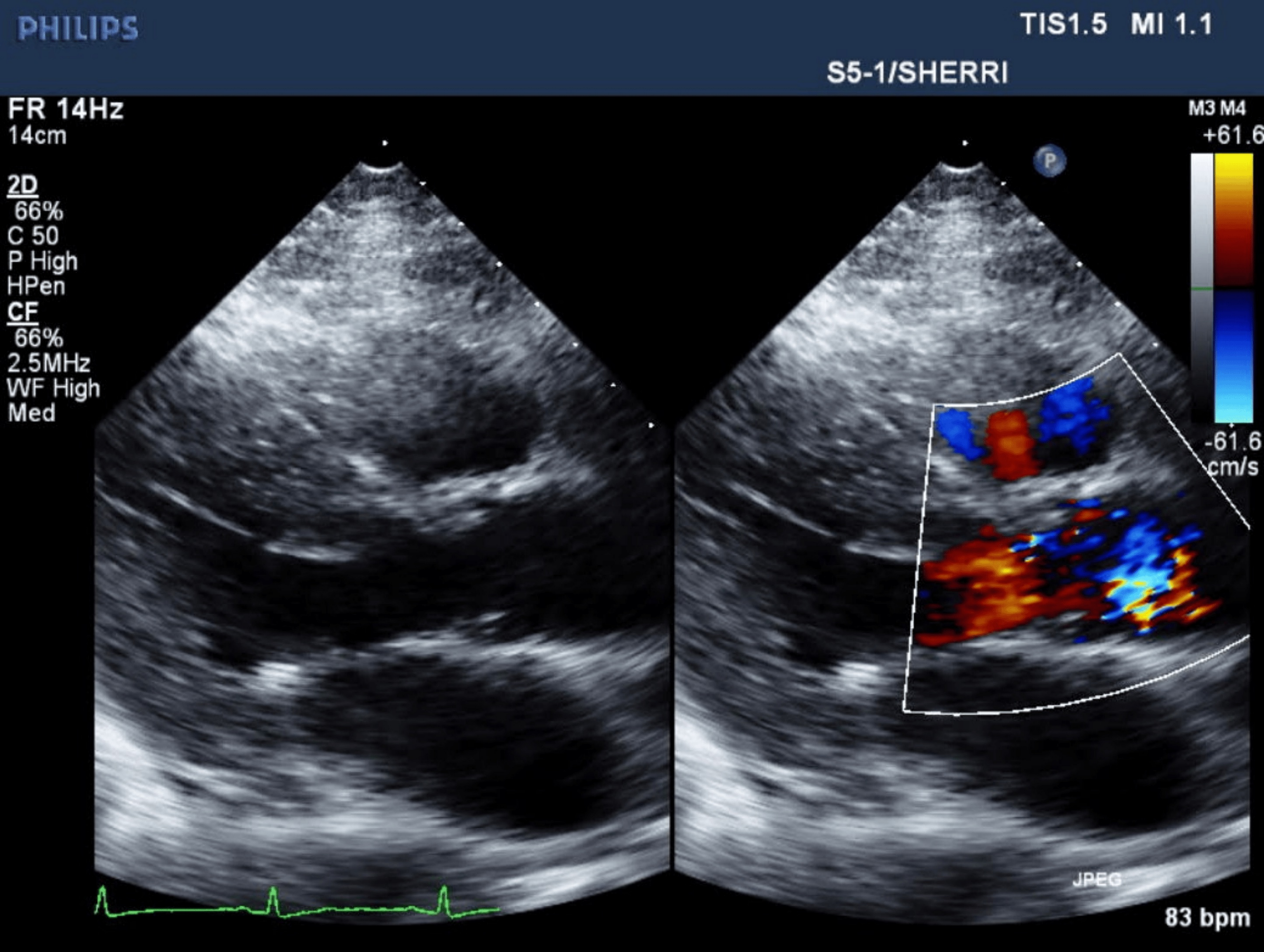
First transthoracic echocardiography since admission with mitral valve visualization without any vegetation
